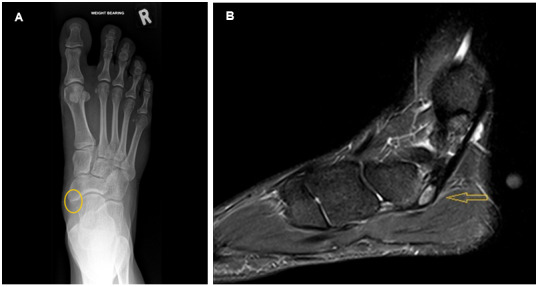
A: Dorsoplantar X-ray view demonstrates the ossicle (yellow circle). B: MRI showing oedematous accessory navicular bone (yellow arrow) and oedema of the medial aspect of the navicular bone.
